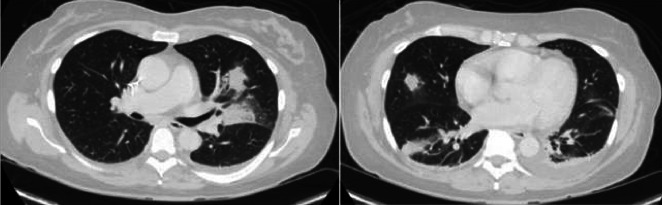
Computed tomography of the chest demonstrating multiple pulmonary opacities consistent with pulmonary septic emboli.
